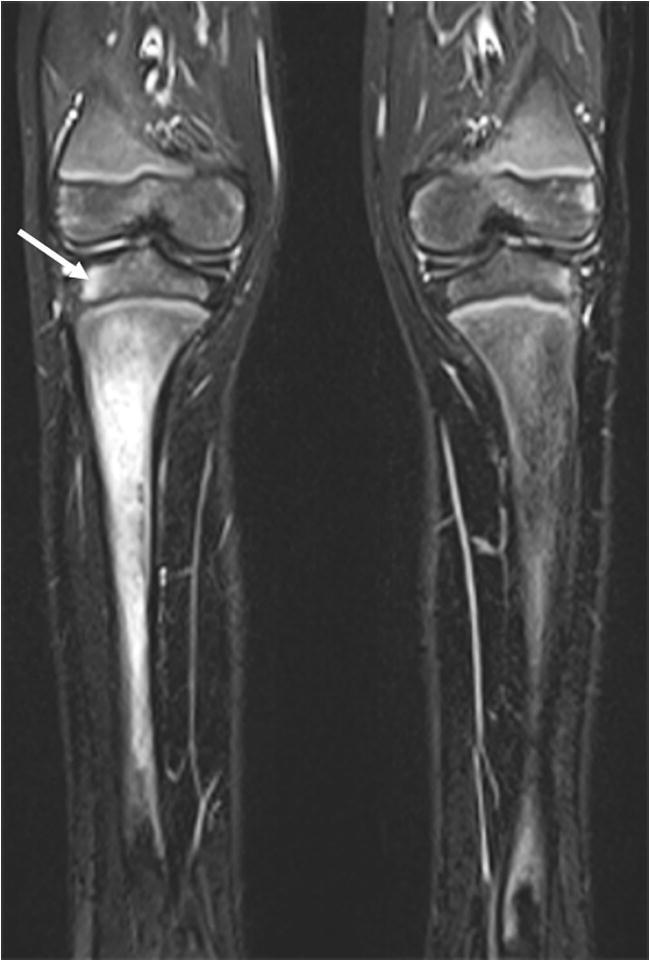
Chronic nonbacterial osteomyelitis in an 8-year-old boy. Coronal T2-W short tau inversion recovery MR image (repetition time/echo time = 5,000/58 ms) of the knees/legs shows involvement of the right tibial metaphysis and diaphysis, and the right epiphysis (*arrow*). The findings were confirmed on T1-weighted images
